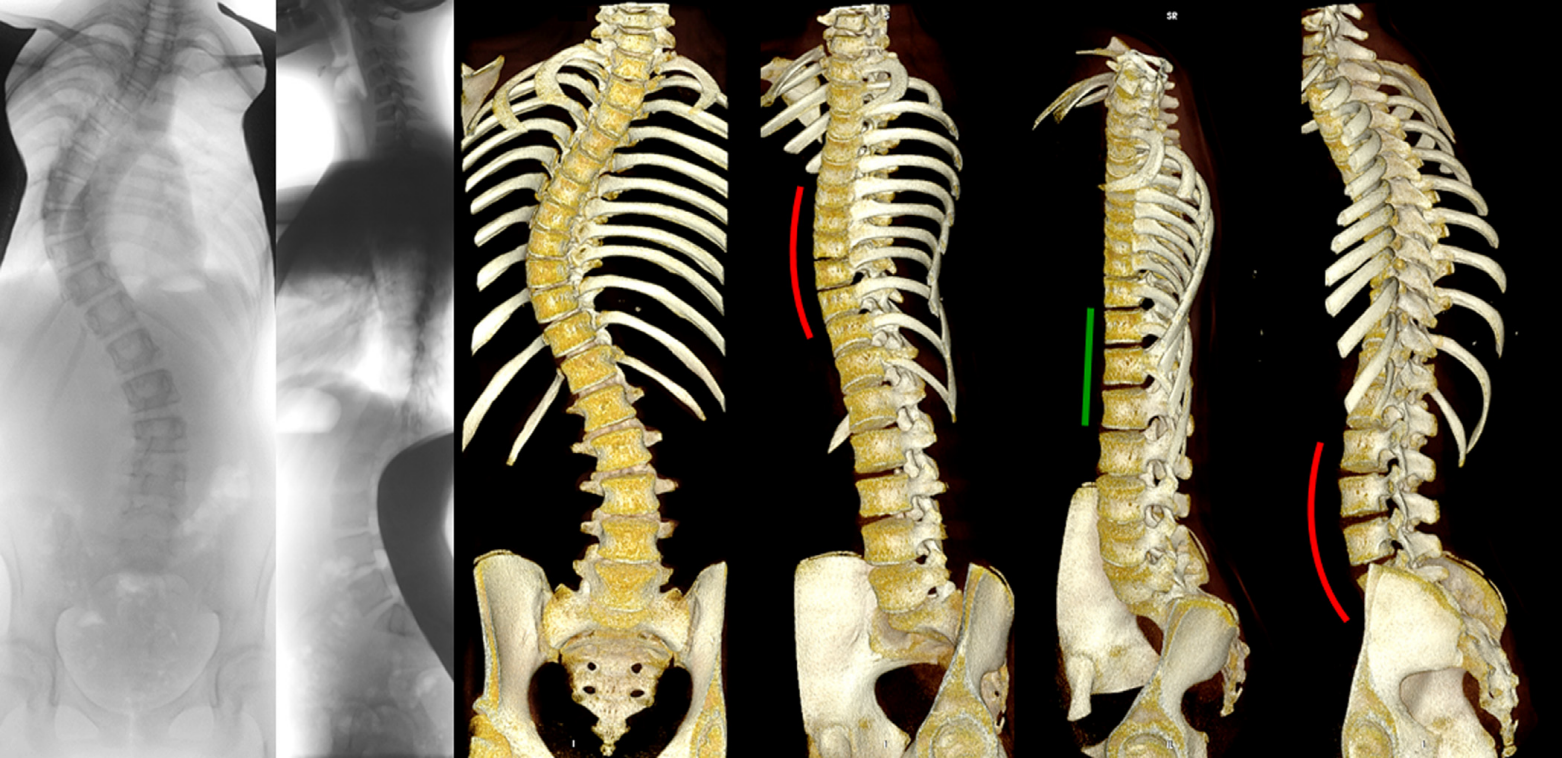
Posterior-anterior and lateral radiographs, and computed tomography reconstructions (OsiriX 64-bit; OsiriX Foundation, Los Angeles, CA, USA) are shown from an anterior and anterolateral view. In this representative 15-year-old patient with adolescent idiopathic scoliosis with a typical thoracic curve pattern, anterior overgrowth cannot be observed on the lateral radiography. The rotated lordoses of both thoracic and the (thoraco)lumbar curvatures, with a clearly longer anterior spinal column than posterior elements, are shown in *red*, and the straight junctional segment in-between in *green* from a true lateral view for these regions.
